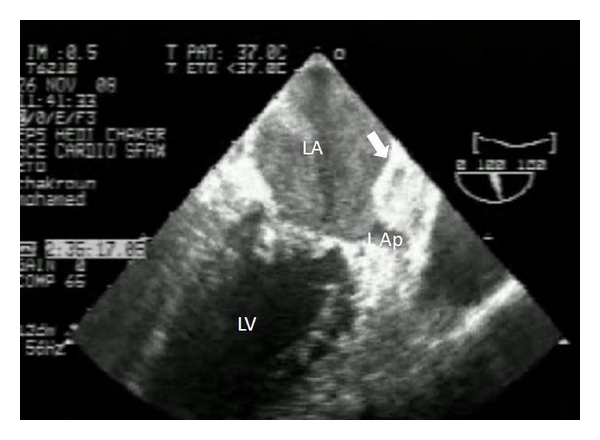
Transesophageal echocardiography showing an important spontaneous contrast and a large mass (white arrow) in both left atrium and appendage (LA: left atrium; LV: left ventricle; L Ap: Left appendage).
